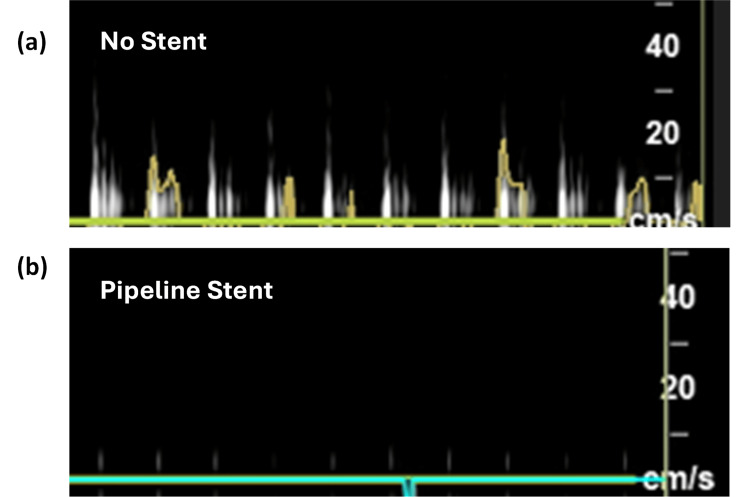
Doppler flow waveforms comparing pulsatility with no stent versus Pipeline stent Doppler flow characteristics in the blood vessel model after deploying the Pipeline stent (Figure 5b) versus no stent control (Figure 5a). A reduction in Vmax, PI, and EDV is seen, with near resolution of pulsatile flow. Waveforms were obtained by utilizing the ultrasound in Doppler mode. All recordings in this figure were taken directly over the targeted stented area. The y-axis represents velocity in cm/s. cm: centimeter, mm: millimeter
